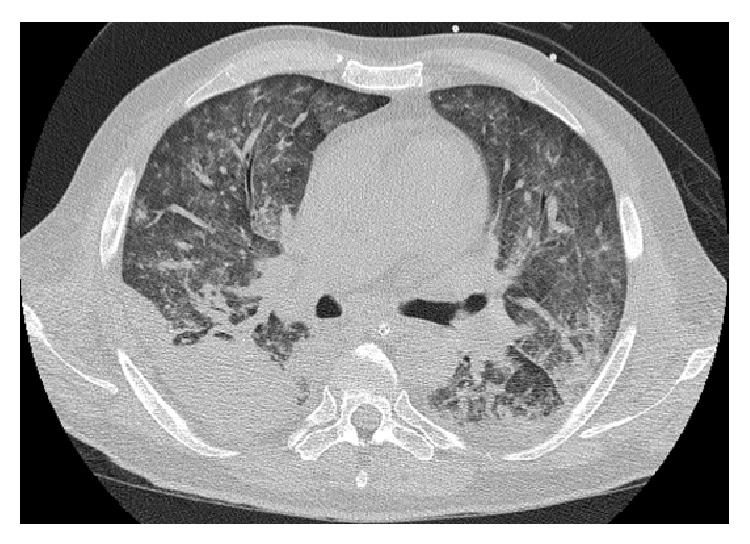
Chest CT scan demonstrating diffuse ground glass opacification with consolidation and pleural effusion. On autopsy, the lungs showed evidence of haemorrhagic infarction in keeping with the CT appearance.
